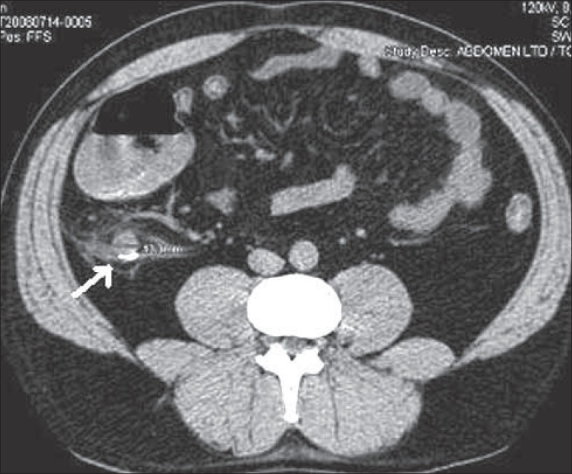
Stump appendicitis. This patient is status post-appendicectomy. Plain CT scan shows inflammatory changes in the right lower quadrant with a dilated tubular structure (arrow)
